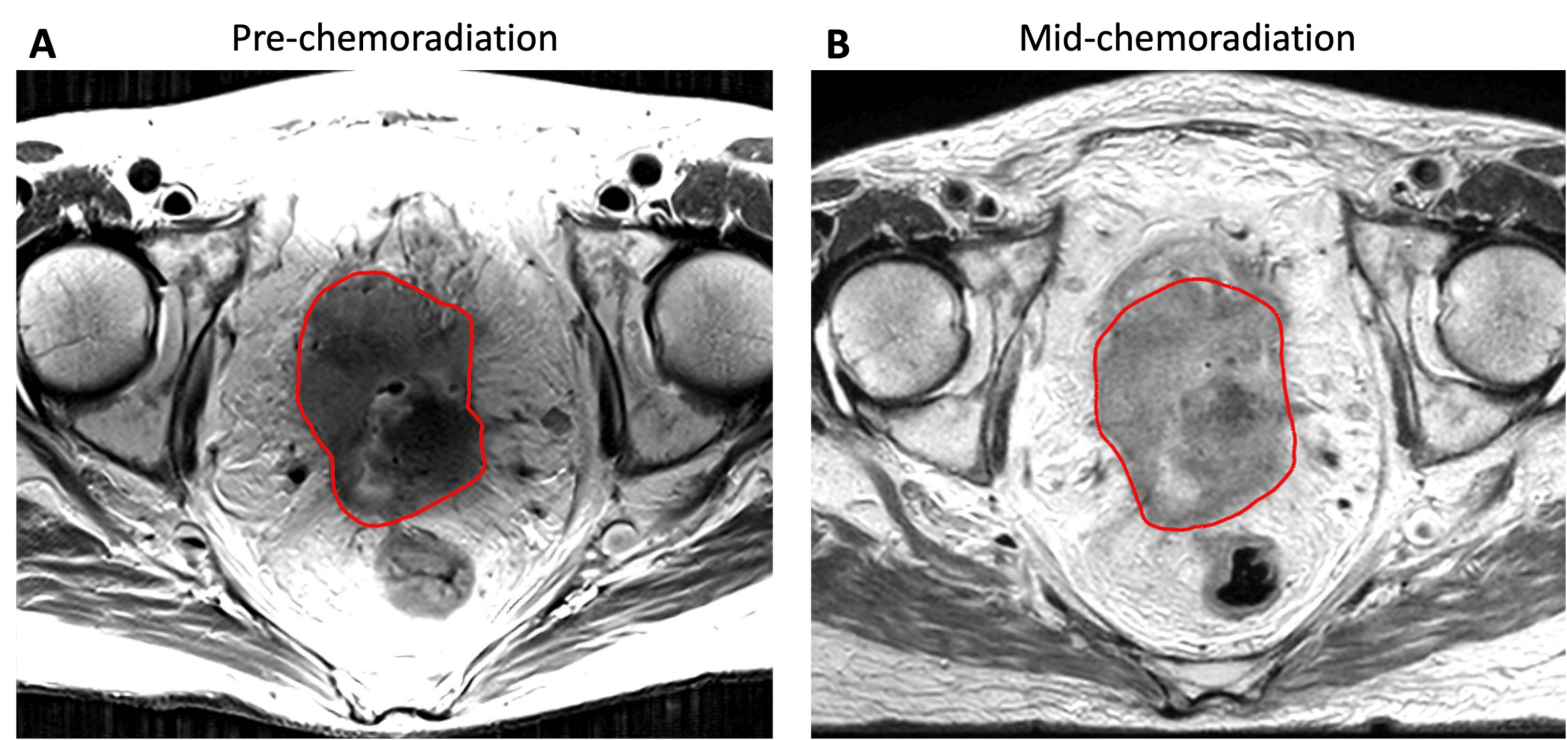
Pre- and mid-treatment T2-weighted MRIs Diagnostic MRI of the tumor pre-treatment (panel A) and MRI of the tumor midway through chemoradiation, prior to boost delivery (panel B), with a red outline indicating the contours used to delineate tumor extent in planning initial external beam (panel A) and boost (panel B) treatments. This patient had minimal response to chemotherapy and external beam radiation. Note the bulky, largely anterior disease, which made this patient ineligible for brachytherapy per the treating gynecologic radiation oncologist. MRI: Magnetic resonance imaging
